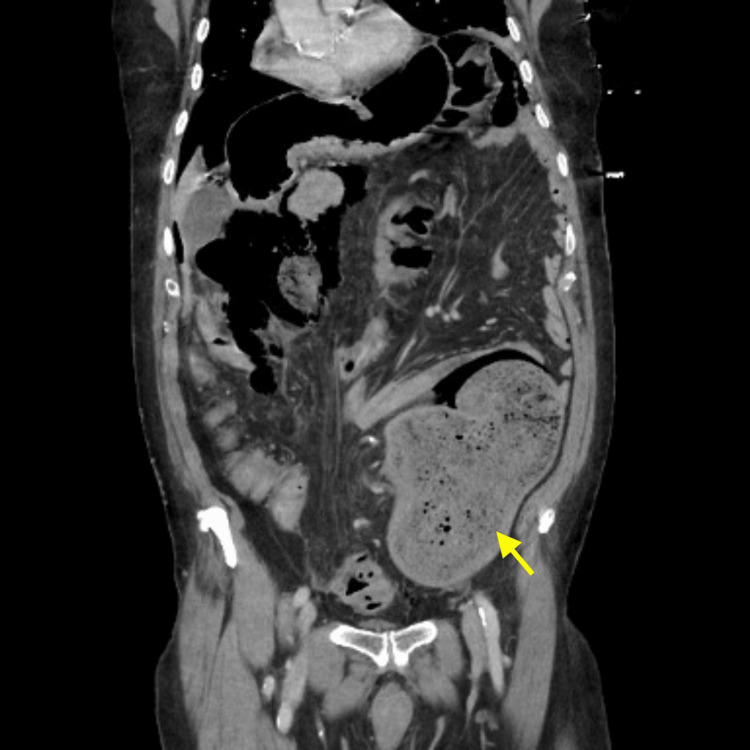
Abdominal/pelvic CT with intravenous contrast, coronal section demonstrating dilatation of the distal descending colon up to 10.5 cm that tapers in the mid-sigmoid region, concerning for sigmoid volvulus CT, Computed Tomography
